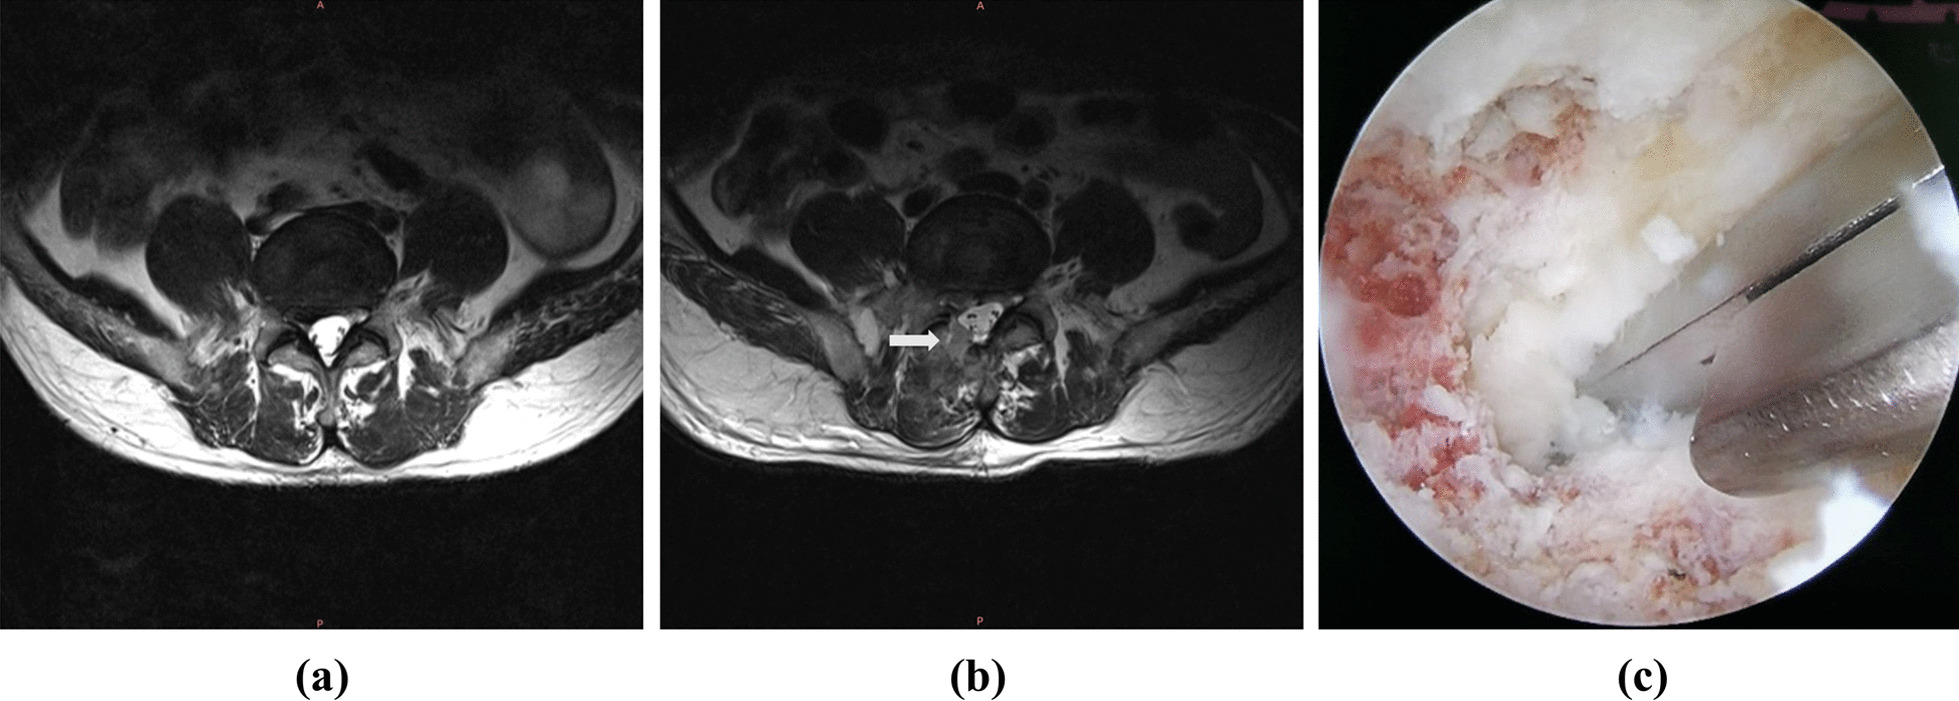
Preoperative and postoperative MRI and intraoperative images of the patient. a Preoperative magnetic resonance axial image of the patient showing a herniated lumbar disc compressing the dural sac. b Postoperative magnetic resonance axial image of the patient showing the patient’s occluded bone. c Intraoperative endoscopic surveillance of the occluded bone
